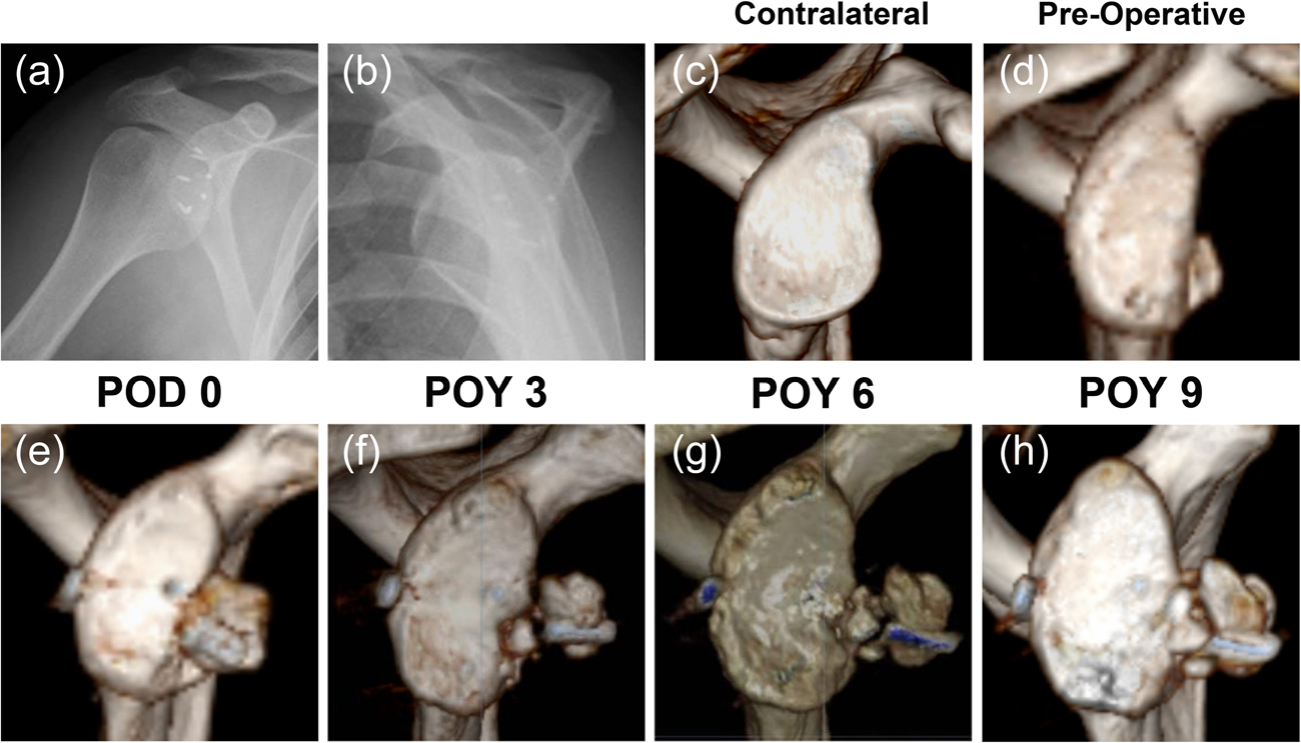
One case (No. 9) of Latarjet revision after Bankart failure of X‐ray (a,b) and 3D CT en face view (c–h). (a,b) The anteroposterior and scapular Y view X‐ray of twice Bankart repair surgeries before he undertook the Latarjet procedure. The bright signal showed the seven metal anchors left over from the previous surgery. (c) Preoperative mirror image of the normal side. (d) Preoperative injury side. (e) The CG‐glenoid interface at POD 0. (e–g) The CG‐glenoid in each follow‐up time point. 3D, three‐dimensional; CG, coracoid graft; CT, computed tomography; POD, postoperative day; POY, postoperative year.
